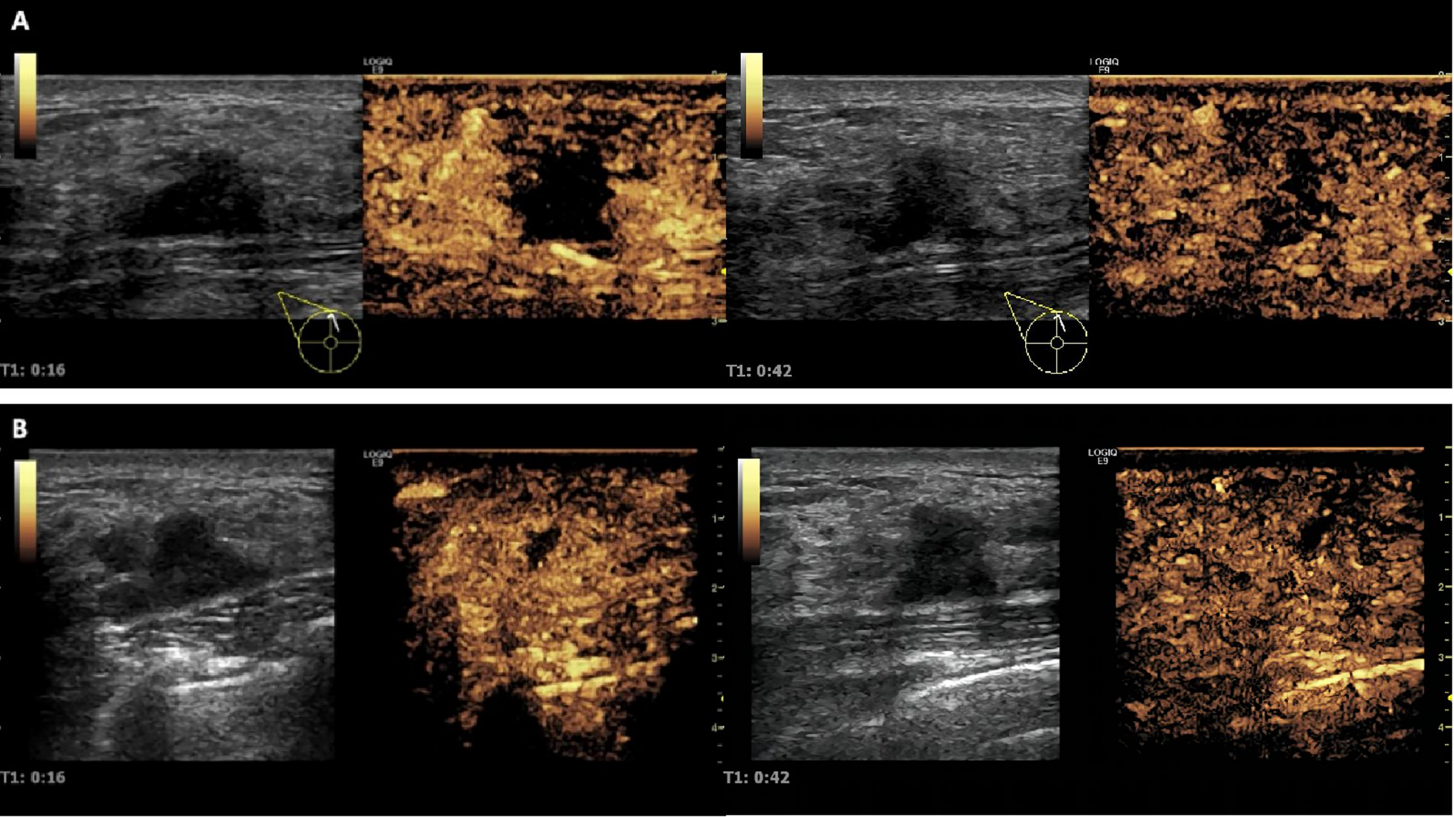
Changes in tumor blood perfusion revealed by CEUS before and after ultrasonic therapy. (A) Before ultrasound treatment, CEUS showed a large perfusion defect area in the lesion; (B) After ultrasound treatment, CEUS showed that the intrafocal perfusion defect area was significantly reduced, and tumor blood perfusion increased significantly.
